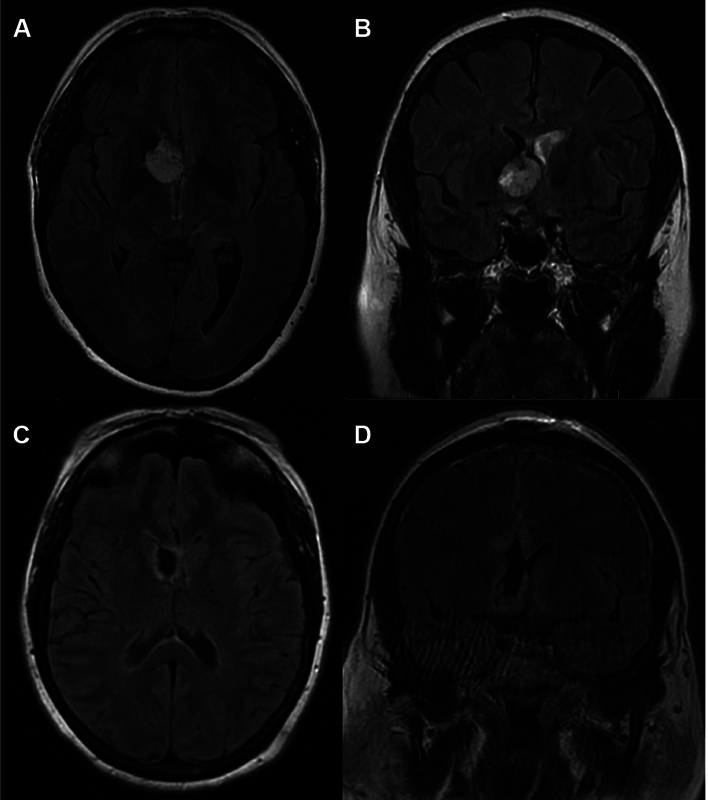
Preoperative axial (A) and coronal (B) contrast-enhanced T2-weighted FLAIR MRI demonstrating the 2.4 mm enhancing mass in the region of resolved right frontal periventricular hemorrhage. Also pictured: postoperative axial (C) and coronal (D) contrast-enhanced T2-weighted FLAIR MRI demonstrating the resection cavity following successful removal of the mass with residual T2/FLAIR hyperintensity most prominent along the posterior resection cavity margin.
